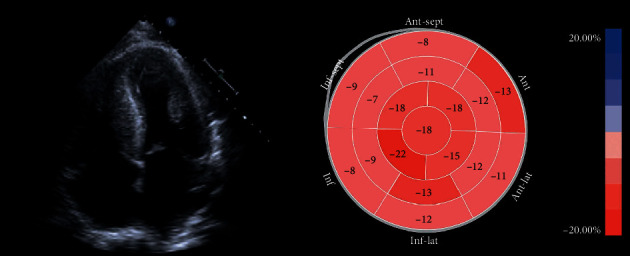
Echocardiography shows low global longitudinal cardiac strain (−13.4%) with “cherry on top” pattern (normal value for global longitudinal strain is −20% and below in healthy individuals). Strain pattern is suggestive of possible cardiac amyloidosis.
